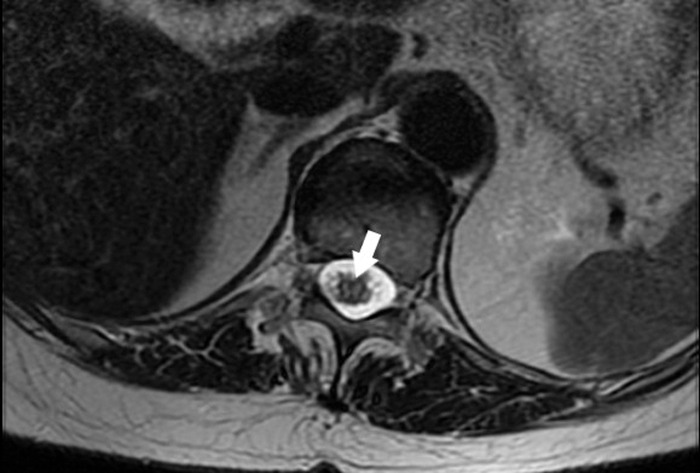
Axial T2-weighted image at the T12 level 14 months postembolization shows mild persistent hyperintense signal with resolved expansion of the central cord (arrow).
